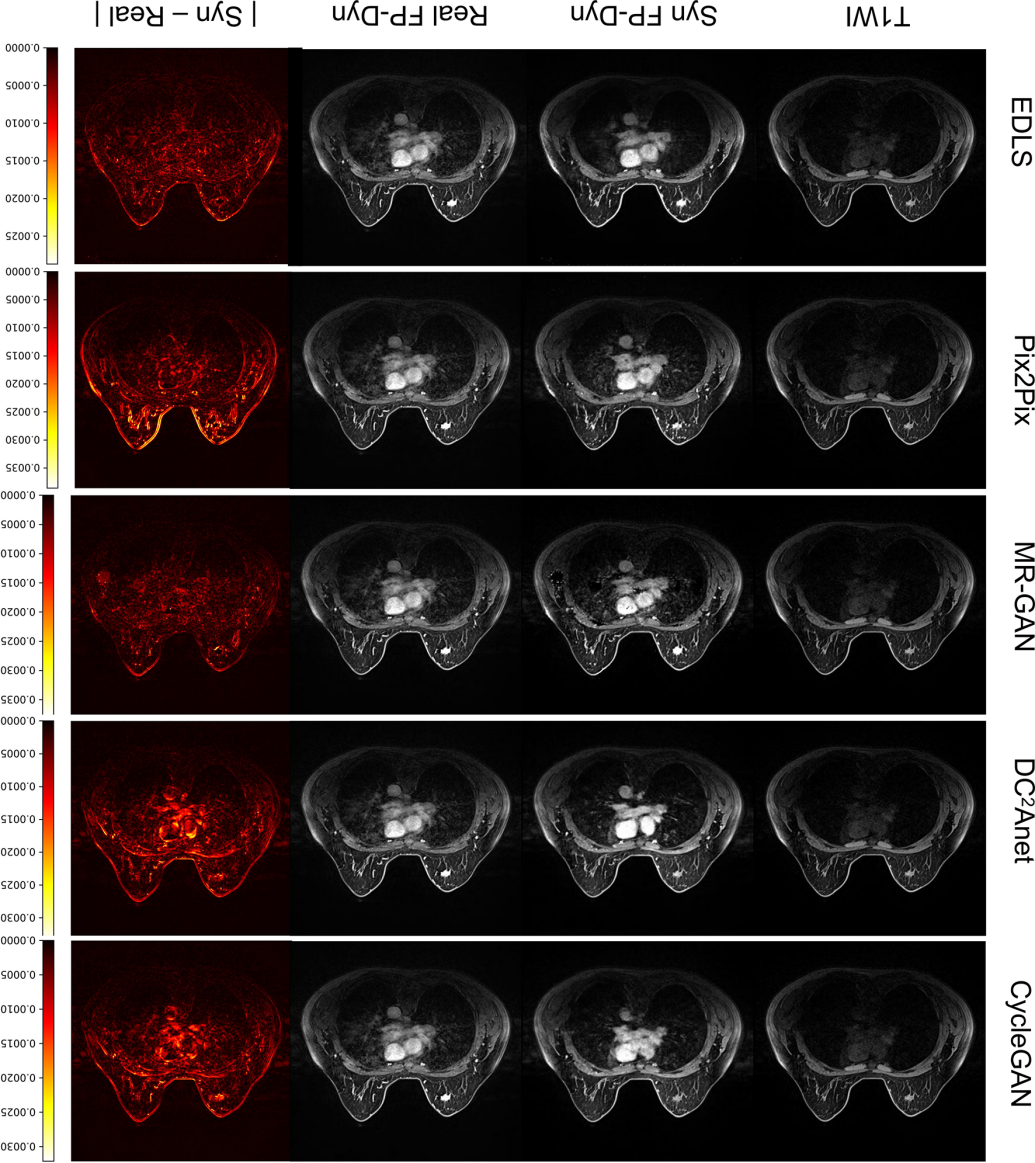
Sample images of the T1WI images, synthesized FP-Dyn images, original FP-Dyn images, and absolute error images. From left to right: T1WI breast MR images, synthesized FP-Dyn images, original FP-Dyn breast MRI, and absolute error images.
